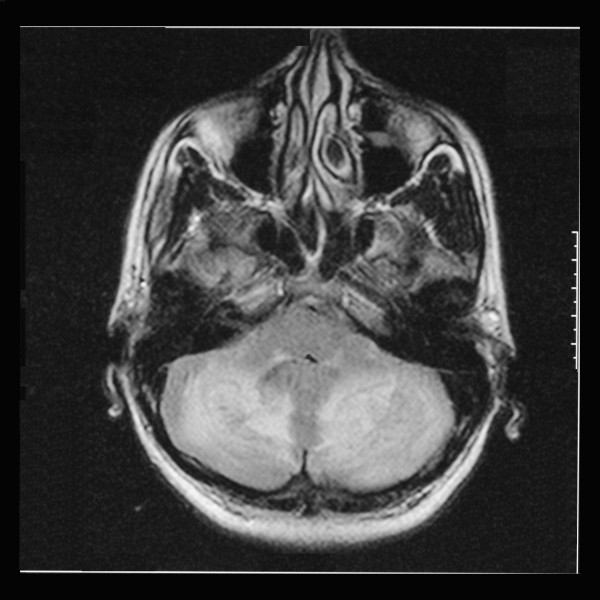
Axial magnetic resonance imaging showing hyperintense areas in his cerebellar white matter and ventricular enhancement in a T2-weighted sequence.
